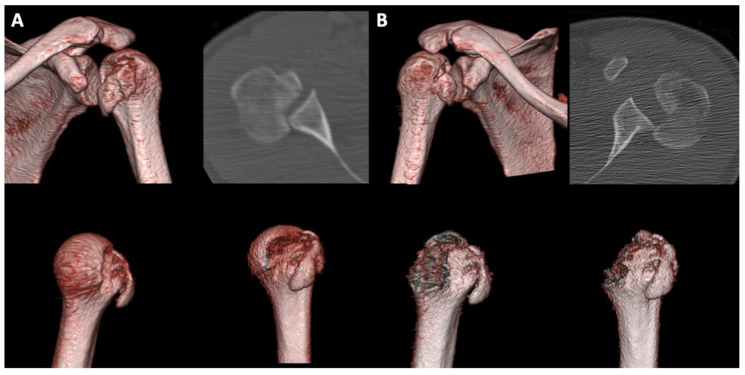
Preoperative imaging of patient from Figure 1; (A) preoperative axial CT-scan and 3D-VRT of the left glenohumeral joint; (B) preoperative axial CT-scan and 3D-VRT of the right glenohumeral joint.
